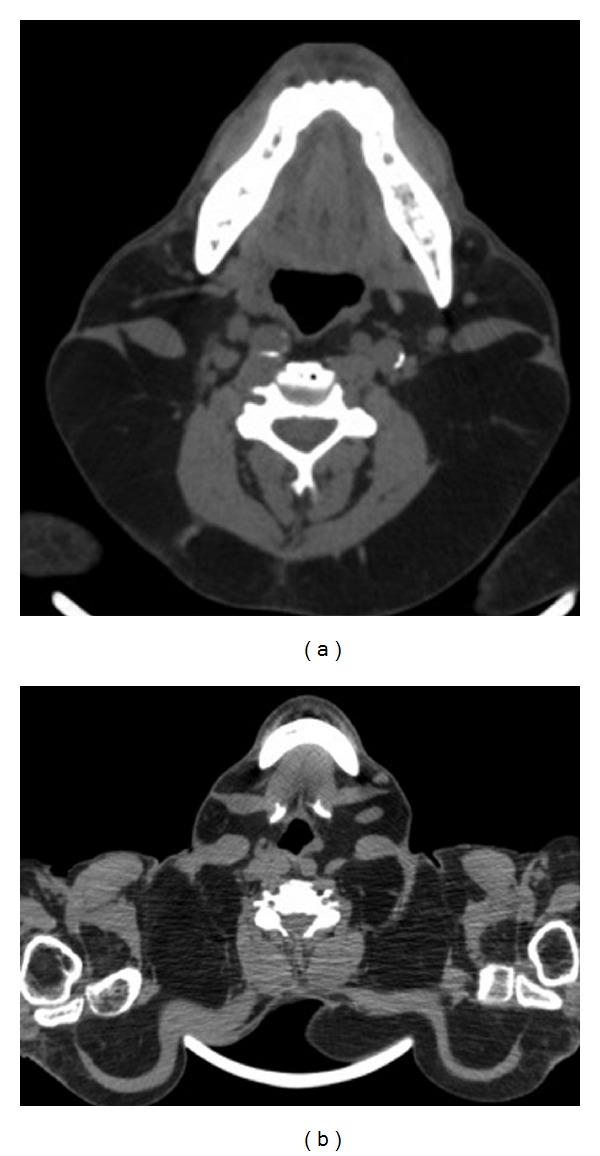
A 53-year-old man with Madelung's disease presenting a progressive painless increase of the cervical region. CT of the neck and chest showed fat deposition occurring predominantly in the posterior subcutaneous region of the neck (a) and in the supraclavicular and upper regions of the chest (b).
